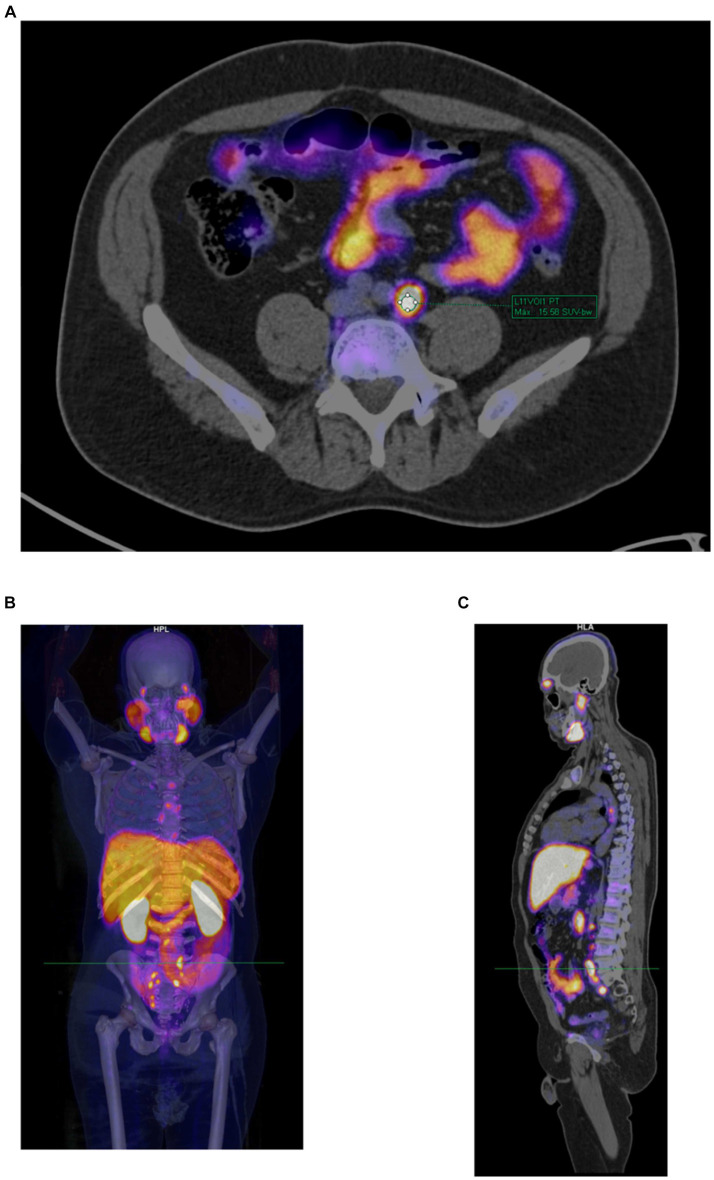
Example of a PET/CT scan with [^18^F]PSMA-1007 in a 53-year-old patient with prostate cancer and post-prostatectomy who had suspected recurrent disease. (A) Manual determination of [^18^F]PSMA-1007 uptake in a lymph node according to the semiquantitative SUVmax (=15.58) using the volume of interest (VOI) tool. (B) Volumetric reconstruction used to show the anatomical site of the evaluated lesion. (C) Sagittal section showing the anatomical site of the evaluated lesion.
